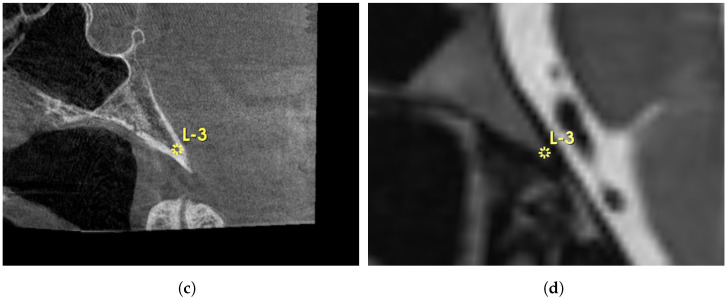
Exemplary overview of a landmark defined in 3DSlicer (yellow “L-3”) in axial (a) and sagittal view (b). As the Euclidean distance of the landmarks increases, the transformation becomes more accurate. Bilaterally, the tip of the anterior semicircular canals was also chosen. The high-resolution DynaCT is depicted in (a,c), whereas the T2-weighted MRI is shown in (b,d).
